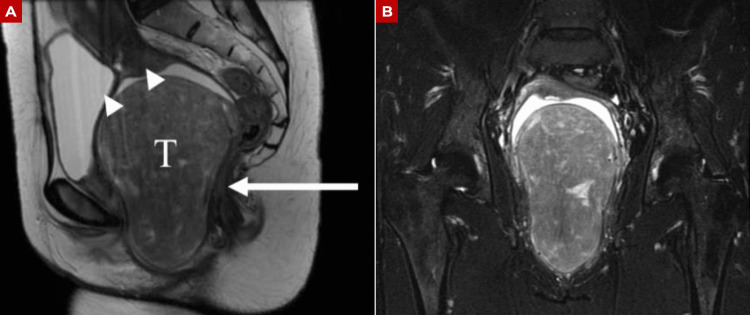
Pelvic magnetic resonance imaging demonstrating a large rectouterine mass with vaginal involvement (A) Sagittal T2-weighted image showing a large, heterogeneous mass occupying the rectouterine space, with loss of the normal interface with the posterior vaginal wall (arrowheads). The lesion (T) extends inferiorly toward the vaginal canal and displaces adjacent pelvic structures, including the uterus and bladder (arrow). (B) Coronal short tau inversion recovery (STIR) image demonstrating a well-defined, hyperintense mass occupying the central pelvis and extending toward the vaginal compartment, suggestive of a lesion with mixed soft tissue and adipose components.
